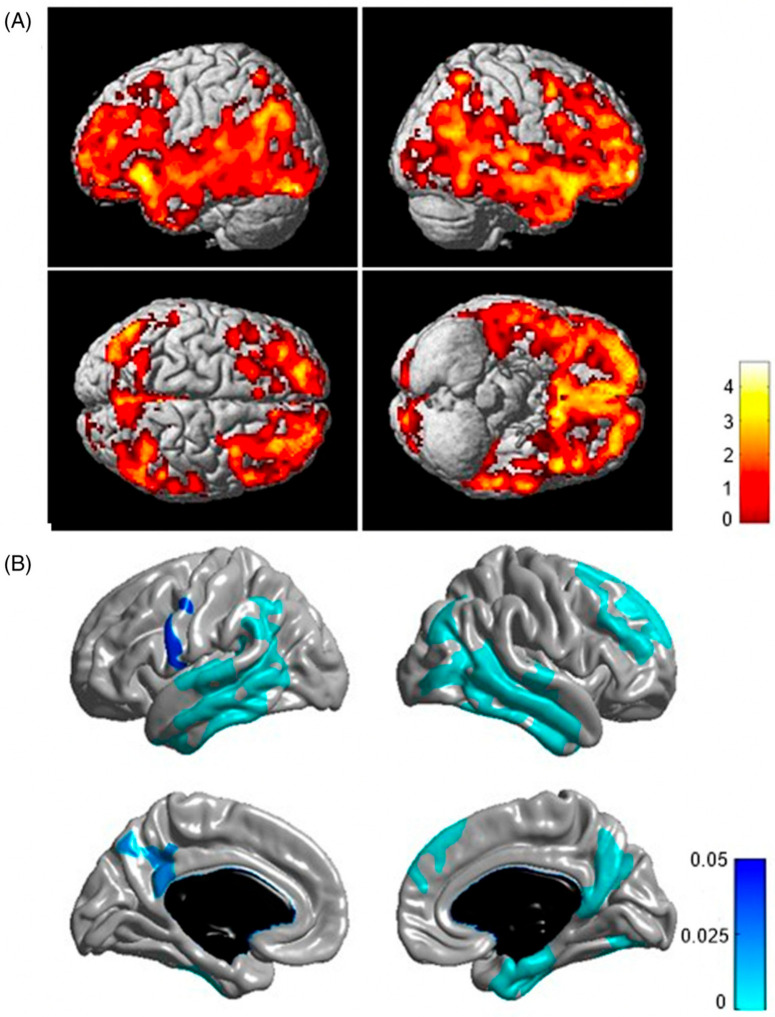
A whole-brain analysis conducted in the ADNI cohort assessed the association between rs10751647 and both (A) amyloid burden (via amyloid PET) and (B) cortical thickness (via MRI). Carriers of the minor allele showed reduced amyloid deposition, particularly in the frontal, parietal, and temporal lobes bilaterally, with statistical significance corrected using a false discovery rate threshold of 0.05. In addition, a greater number of minor alleles was linked to increased cortical thickness in the bilateral temporal regions, including the entorhinal cortex. These associations met significance criteria following random field theory correction at *p* = 0.05. Abbreviations: ADNI = Alzheimer’s Disease Neuroimaging Initiative; MRI = magnetic resonance imaging; and PET = positron emission tomography. Image reprinted without changes from Pyun et al. [41] under the Creative Commons Attribution 4.0 International License (CC BY). http://creativecommons.org/licenses/by/4.0/ (accessed on 12 June 2025).
